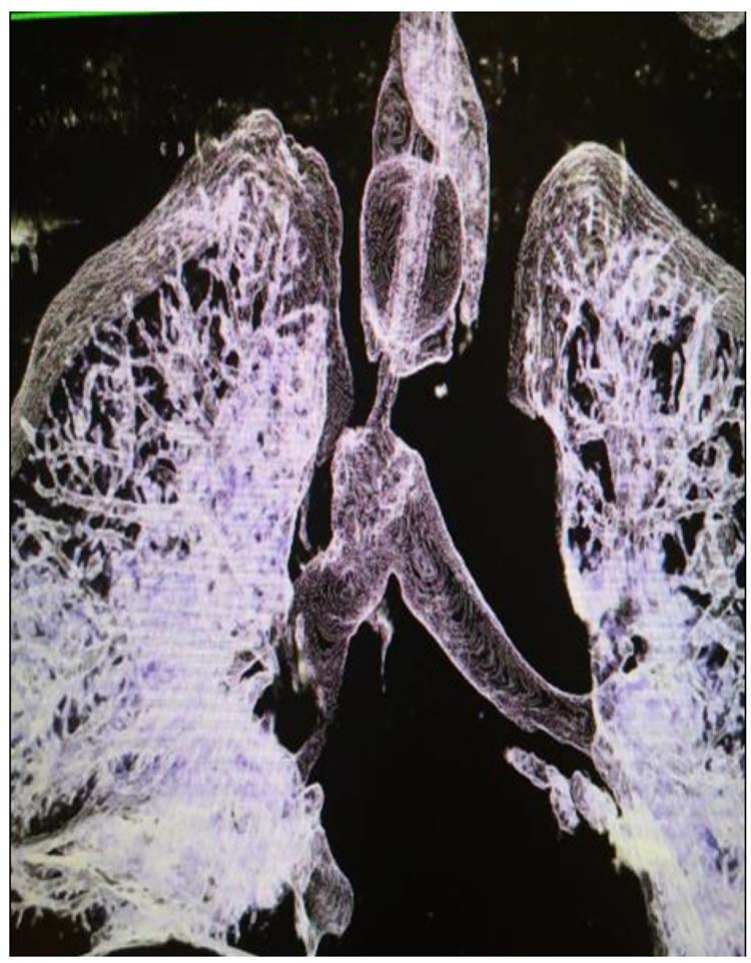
Reconstructed CT scan showing injury site of trachea.
